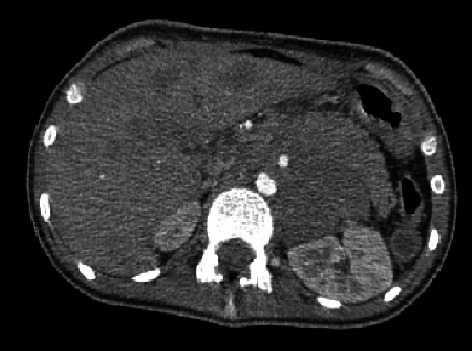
Computerized tomography (CT) scan of mass and metastasis. Large lobulated heterogeneous enhancing mass in the posterior mediastinum with multiple hypodense lesions were seen in both hepatic lobes, suggestive of the metastatic lesion.
